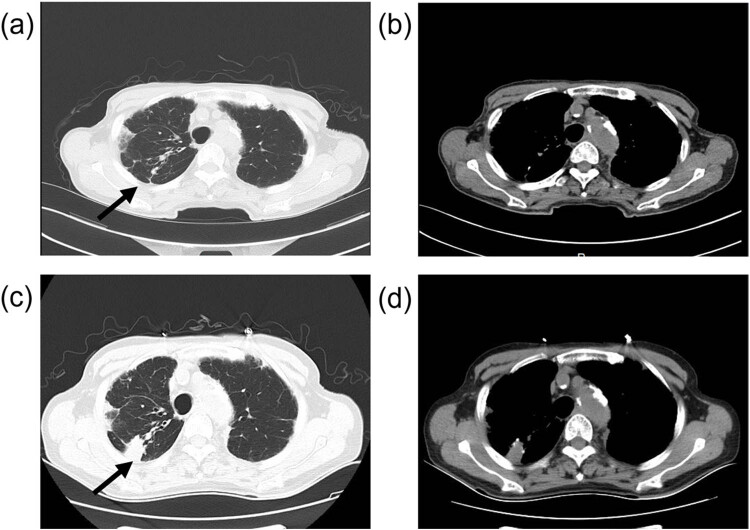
The patient's chest CT scan results from various time points revealed the emergence of new patchy high-density shadows, as indicated by the black arrow, in the upper lobe of the right lung (c) and (d), compared to previous scans (a) and (b).
